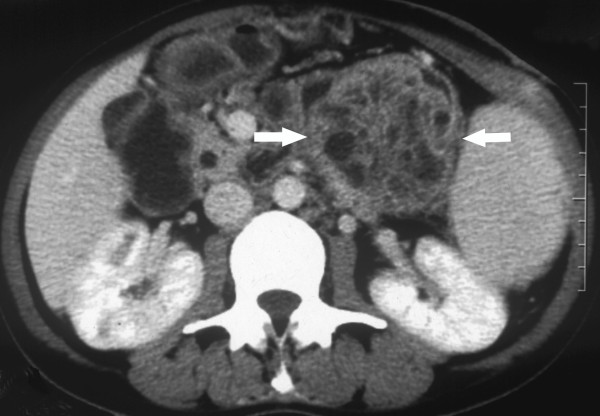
Abdominal CT scan. The tumor showing a heterogeneous multiloculated cystic mass with low density in the tail of the pancreas (white arrow), compressing the adjacent organs.
